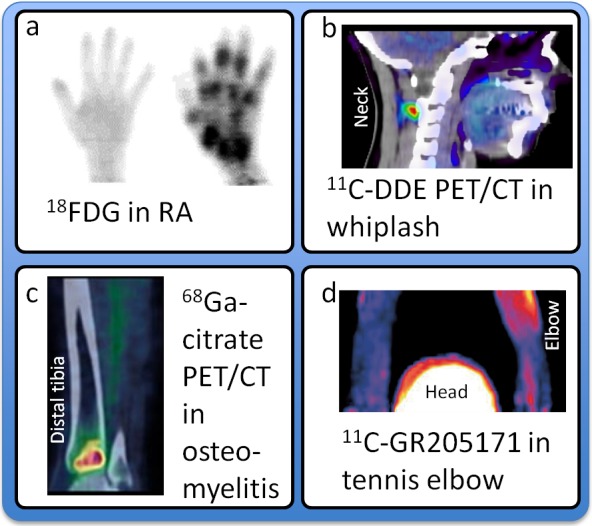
Examples of PET Imaging of Peripheral Pain Mechanisms. A:^18^F-FDG PET of the hand of a healthy subject and a patient with rheumatoid arthritis. Adapted from Beckers et al.^9^ B:^11^C-D-deprenyl PET/CT of a patient with whiplash-associated disorder. Adapted from Linnman et al.^11^ C:^68^Ga-citrate PET/CT of a patient affected by acute osteomyelitis of the left distal tibia. Adapted from Figure 6 (A ^68^Ga-citrate PET/CT scan of a patient affected by acute osteomyelitis of the left distal tibia. The scan demonstrates an area of increased tracer uptake (red area), corresponding to an area of decreased bone density on the CT images, which is consistent with acute inflammation) by Roivainen et al.^16^ with kind permission from Springer Science and Business Media. D:^11^C-GR205171 PET of a patient with unilateral chronic tennis elbow. Adapted from Peterson.^17^
